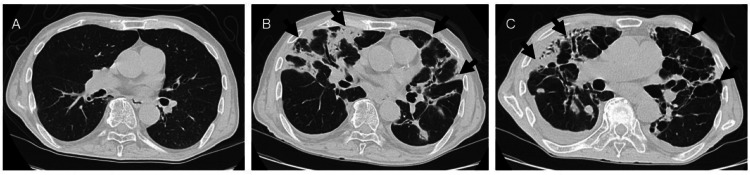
Serial changes on chest CT images. (A) Initially, there were no findings suggestive of pneumonia. (B) Five months later, bilateral diffuse infiltrates developed (arrows), which progressed to bronchiectasis and multiple lung cysts (arrows) eight months after the initial infection (C). CT, computed tomography
